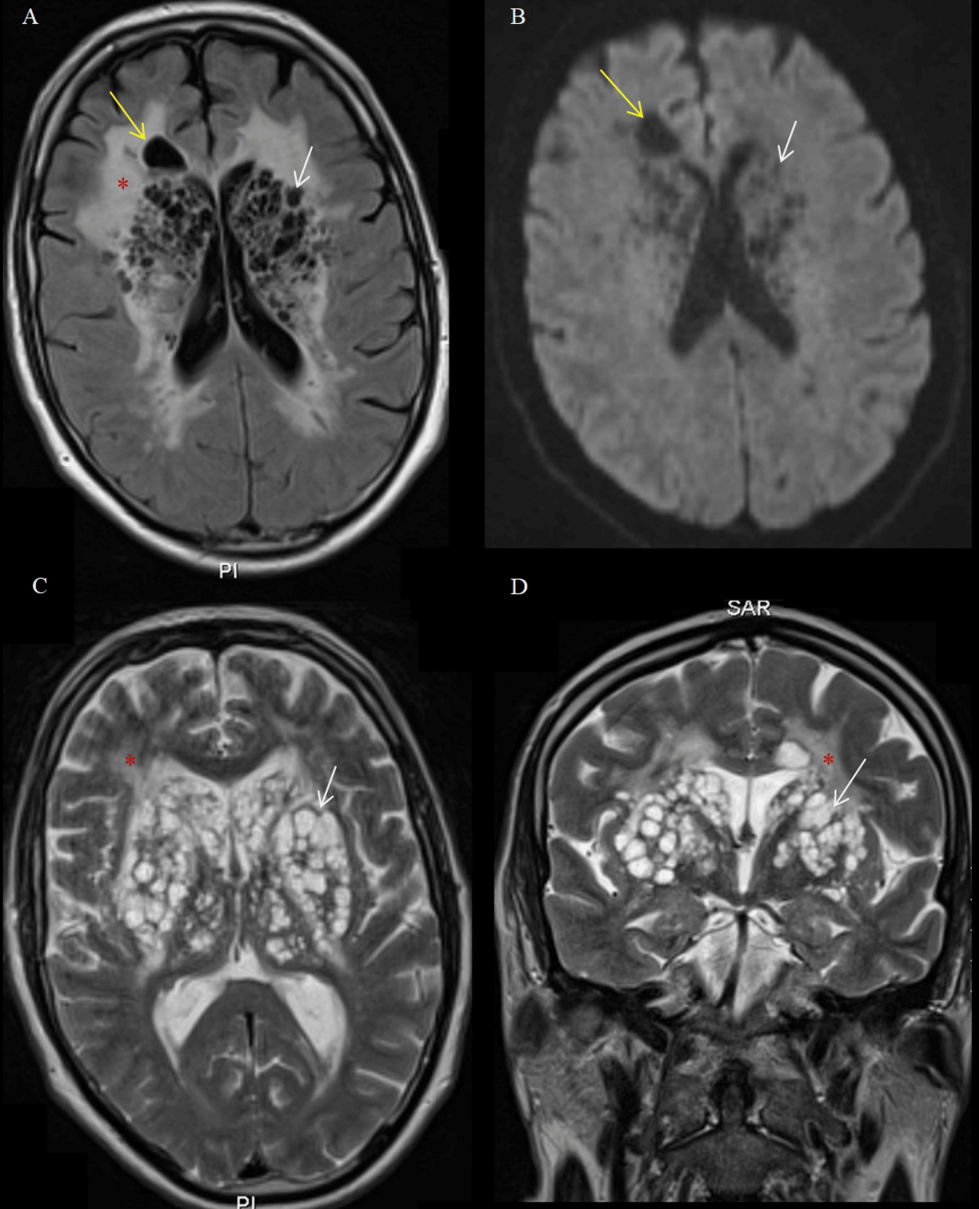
Brain MRI showing bubbly-like cystic lesions, Type I Virchow-Robin space (VRS) dilation, and severe leukoencephalopathy (Fazekas grade 3) (A) Axial FLAIR sequence revealing hypointense CSF-like cystic lesions in the lenticulostriate region (white arrow) and right frontal area (yellow arrow), in association with diffuse periventricular leukoencephalopathy presenting a marked hyperintense signal (red asterisk). (B) DWI sequence demonstrating the absence of diffusion restriction within the cystic lesions in the lenticulostriate region (white arrow) and the right frontal region (yellow arrow). (C) and (D) T2-weighted MRI slices in axial (C) and coronal (D) views demonstrating the corresponding cystic lesions (white arrows) and hyperintense leukoencephalopathy (red asterisk).
